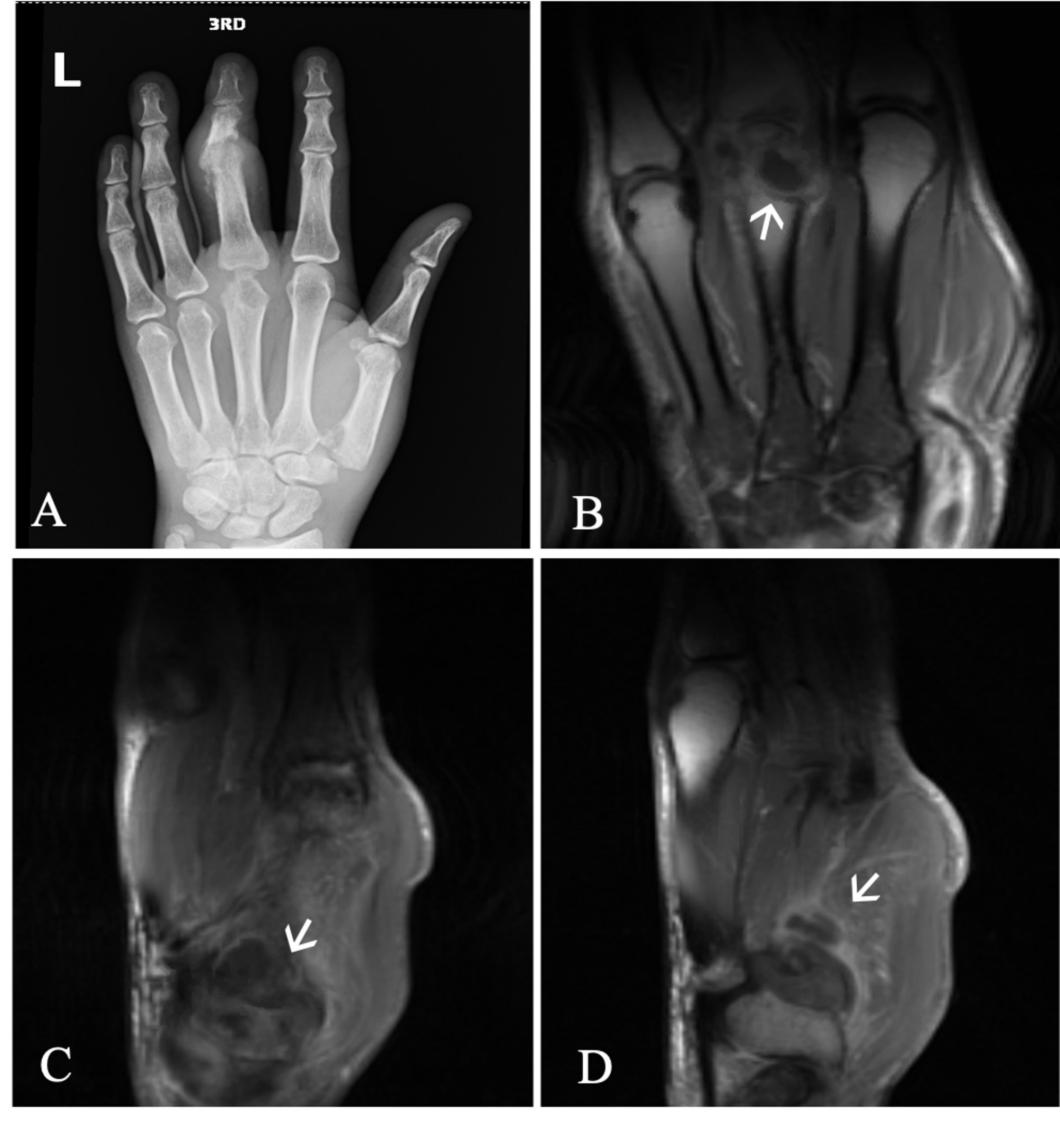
Left hand imaging demonstrates multiple areas concerning for infectious and inflammatory arthritis, tenosynovitis, tophus, intraosseous abscess, and osteomyelitis. (A) Left hand X-ray exhibits multifocal areas of bone erosion, primarily affecting the third metacarpophalangeal (MCP) and proximal interphalangeal joints, base of the thumb, and distal radius. (B) Contrast-enhanced multiplanar, multisequence MRI of the left hand demonstrate the third MCP joint is distended with fluid, T1 hypointense signal and marrow edema in the adjacent metacarpal and proximal phalanx, and a peripherally enhancing fluid collection in the third metacarpal head or intraosseous tophus (arrow) measuring 9 x 12 mm. (C) The first metacarpal and trapezium show diffuse marrow edema and T1 hypointense signal, joint recesses are distended with fluid, erosion of the head of the first metacarpal exists along its ulnar aspect, and a peripherally enhancing focus (arrow) exists in the base of the first metacarpal measuring 10 x 12 mm. (D) Fluid distention of the third MCP joint, third extensor digitorum tendon sheath, and first carpometacarpal joint (arrow) likely represent infectious or inflammatory arthritis and tenosynovitis.
